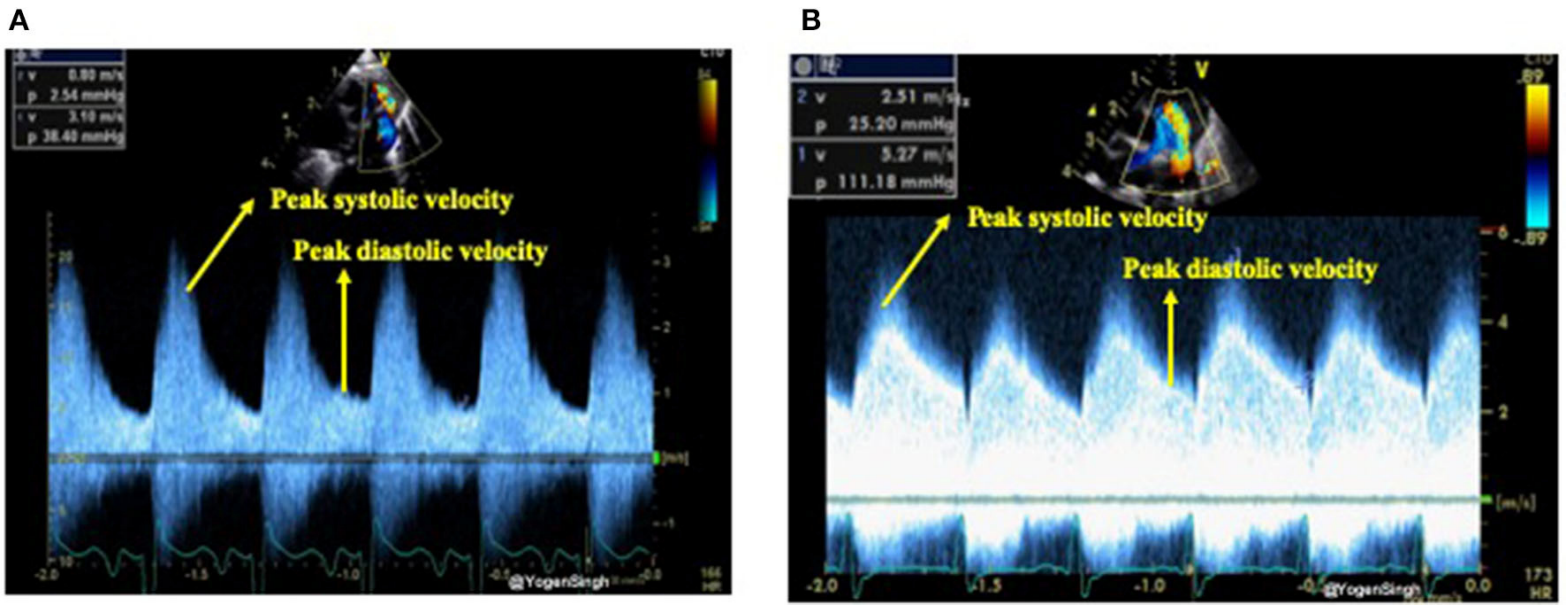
Assessment of restrictive and non-restrictive (pulsatile) flow pattern on Doppler assessment of PDA. (A) Showing non-restrictive (pulsatile) flow pattern with end-diastolic velocity (EDV) less than half of the peak systolic velocity; and (B) showing restrictive flow pattern with end-diastolic velocity (EDV) more than half of the peak systolic velocity.
